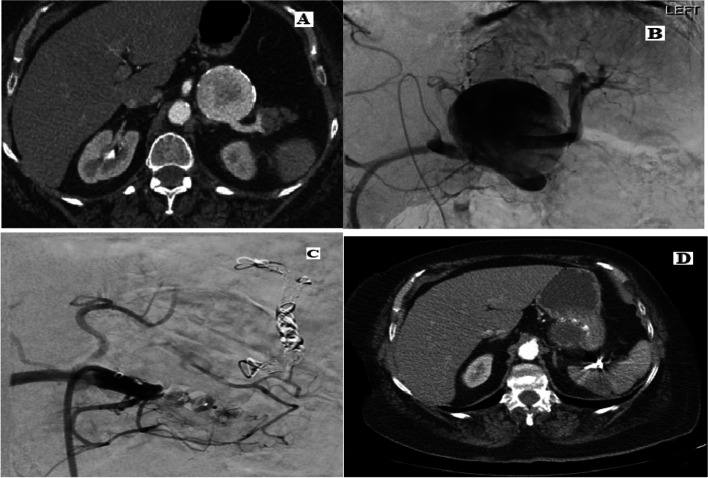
Splenic artery aneurysm managed by front and back door embolisation using vascular plug for the front door and coils for the back door. A Axial arterial phase post contrast CT showing 5.5 cm main artery SAA. B Coeliac angiogram confirms the SAA. C Embolisation using vascular plug for the front door and coils for the back door. D 2 months follow up arterial phase post contrast CT confirming exclusion of the aneurysm from the circulation with less than 20% infarction of the splenic parenchyma without clinical significance
